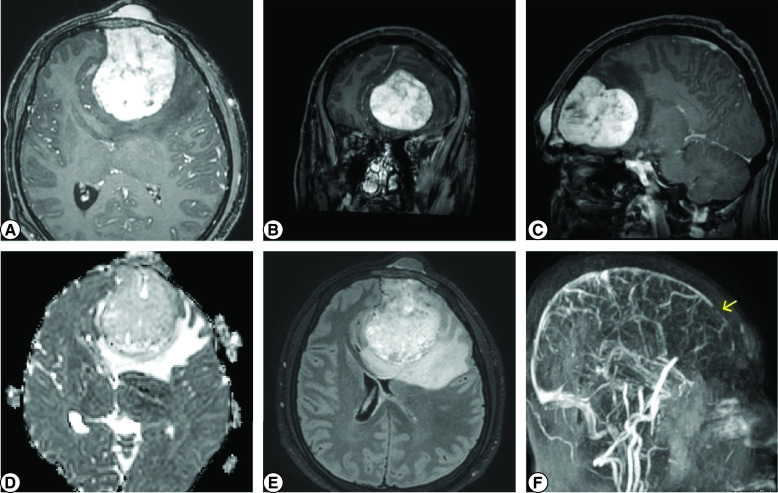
MRI of the brain with contrast. (A) Axial, (B) Coronal, and (C) Sagittal sequences showing large contrast enhancing left frontal mass with osseous infiltration, marked mass effect upon the adjacent brain, subfalcine herniation and marked compression of the frontal horns of the lateral ventricles seen. (D) ADC map sequence showing no restriction. (E) Flair sequence showing associated edema. (F) MRV showing invasion of the anterior part of SSS (arrow). ADC: Apparent diffusion coefficient; FLAIR: Fluid-attenuated inversion recovery; MRV: Magnetic resonance venography; SSS: Superior sagittal sinus.
